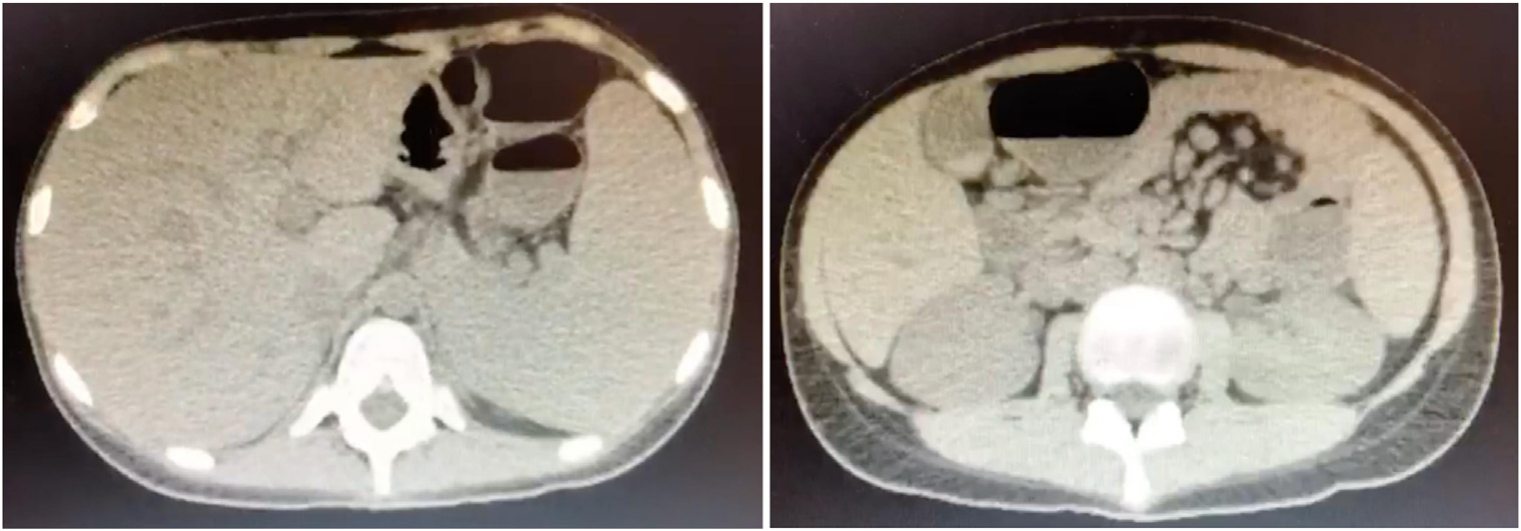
Axial abdominal computed tomography images showing hepatosplenomegaly and enlarged mesenteric and retroperitoneal lymph nodes. Computed tomography images obtained in August 2021 for investigation of severe gastrointestinal symptoms.
